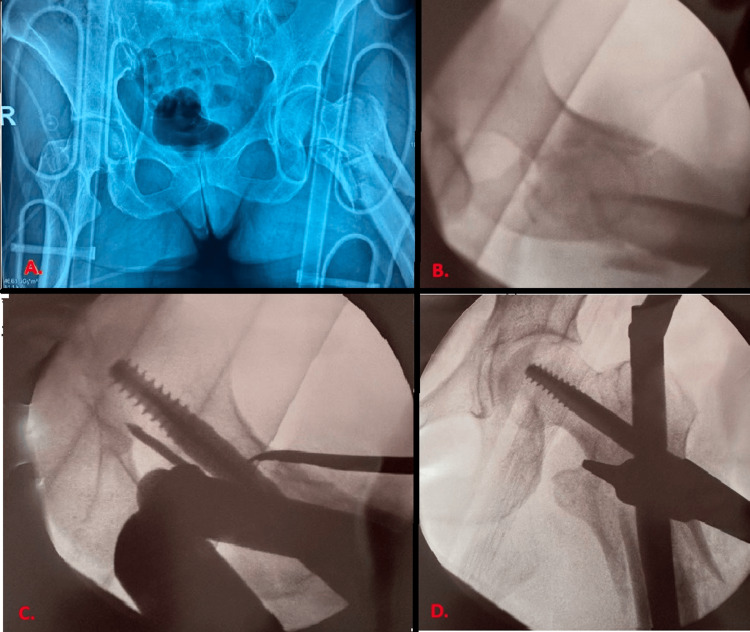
Preoperative and intraoperative radiographs (A) AP radiograph of the pelvis at presentation in the emergency room. (B) Intraoperative C-arm image of the fracture under traction at the start of the index operation. (C, D) Lateral and AP intraoperative C-arm radiographs, respectively, showing femoral neck reduction with a Hohmann retractor and hip screw placement during the same operation. A Steinmann pin was used to prevent femoral head rotation during hip screw insertion.
